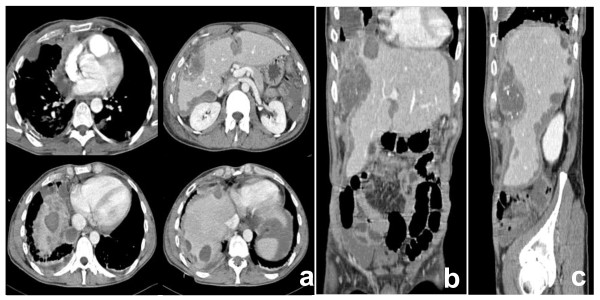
CT appearance of PEComa: axial (a), coronal (b) and sagittal (c) reconstructions. CT of the abdomen showed a 7 cm lesion in the right lobe of the liver with multiple ovular lesions of 0.5-3.5 cm along right triangular ligament, hypoattenuating relative to the surrounding hepatic tissue with minute calcifications. Hypodense lesions along right triangular ligament and capsular profile of falciform ligament were additionally documented. In the thorax CT images, abnormal lymph nodes of 2,8 cm in length were observed along internal right mammary chain, anterior mediastinum and aorta-lung interface. Multiple nodular lesions of the lung were documented in the right hemi-thorax.
